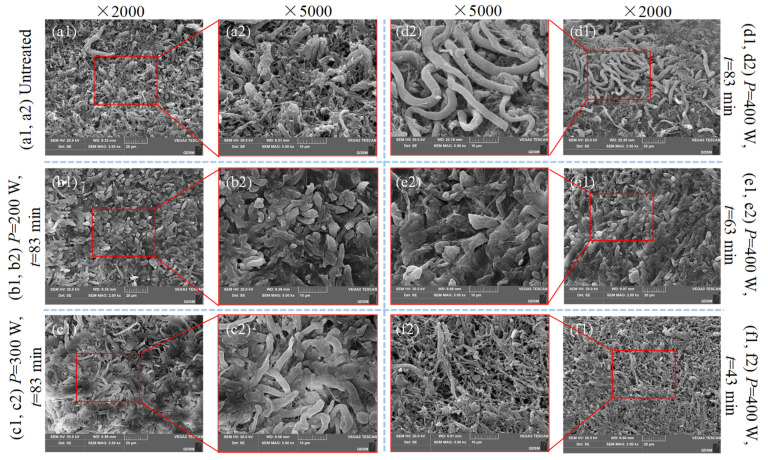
Microstructure of semi-dry salted *Apostichopus japonicus* subjected to different ultrasonic treatments.
